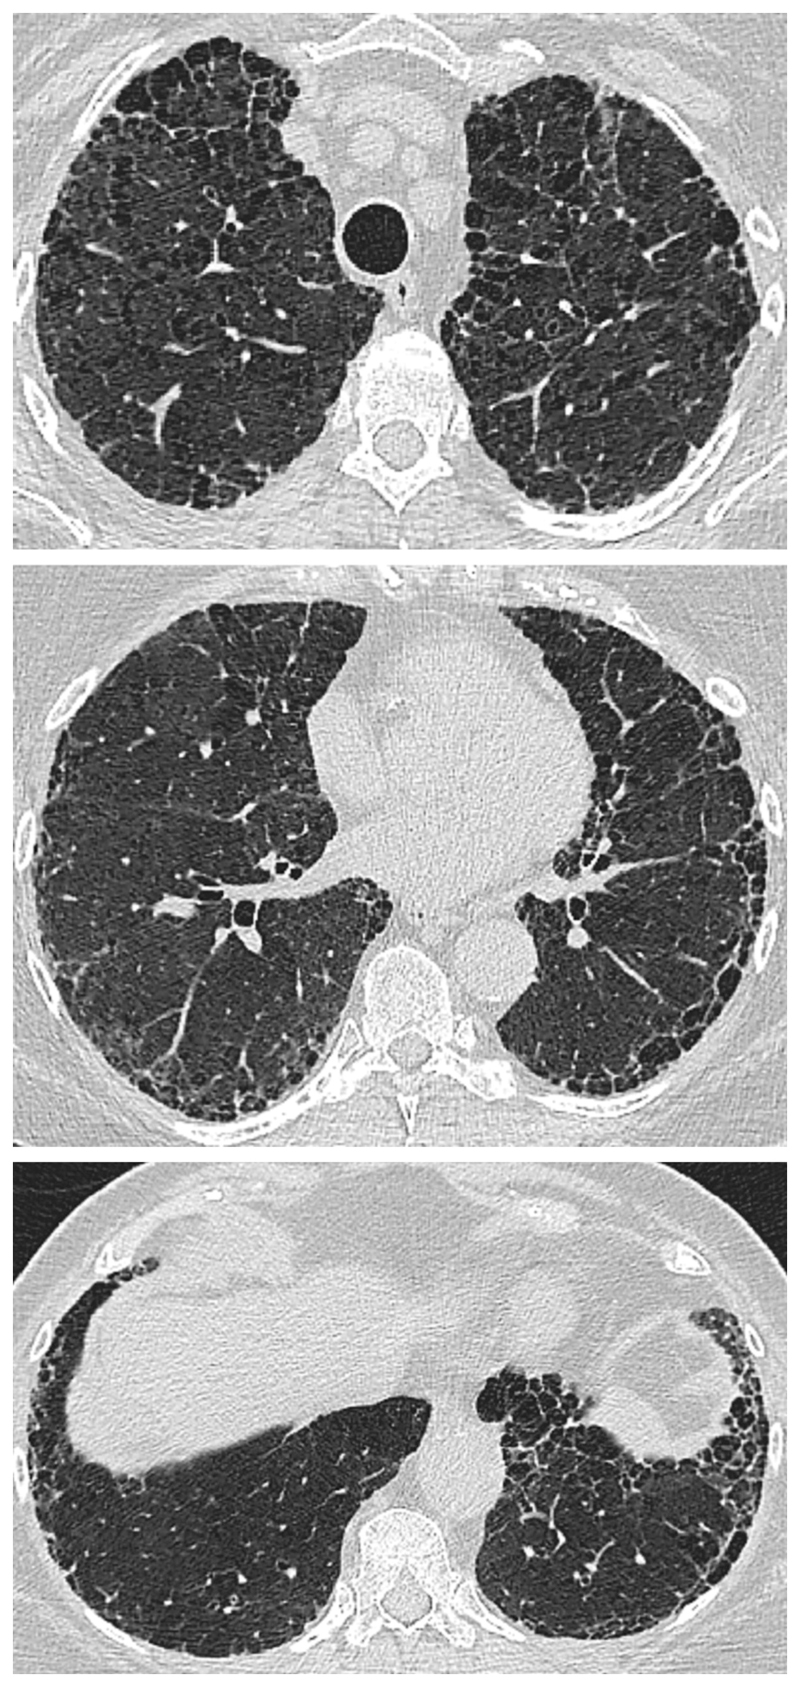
HRCT showing a typical distribution of disease seen in combined pulmonary fibrosis and emphysema (paraseptal emphysema pattern). In this 67 year-old male diagnosed with idiopathic pulmonary fibrosis, extensive isolated paraseptal and centrilobular emphysema is present in the upper zones. Whilst the centrilobular emphysema is mostly isolated in the midzones, paraseptal emphysema is increasingly admixed resembling honeycomb cysts in the left lung. Within the left lower lobe, paraseptal emphysema mimicking honeycomb cysts lies adjacent to more centrally placed irregularly shaped centrilobular emphysema (arrow).
